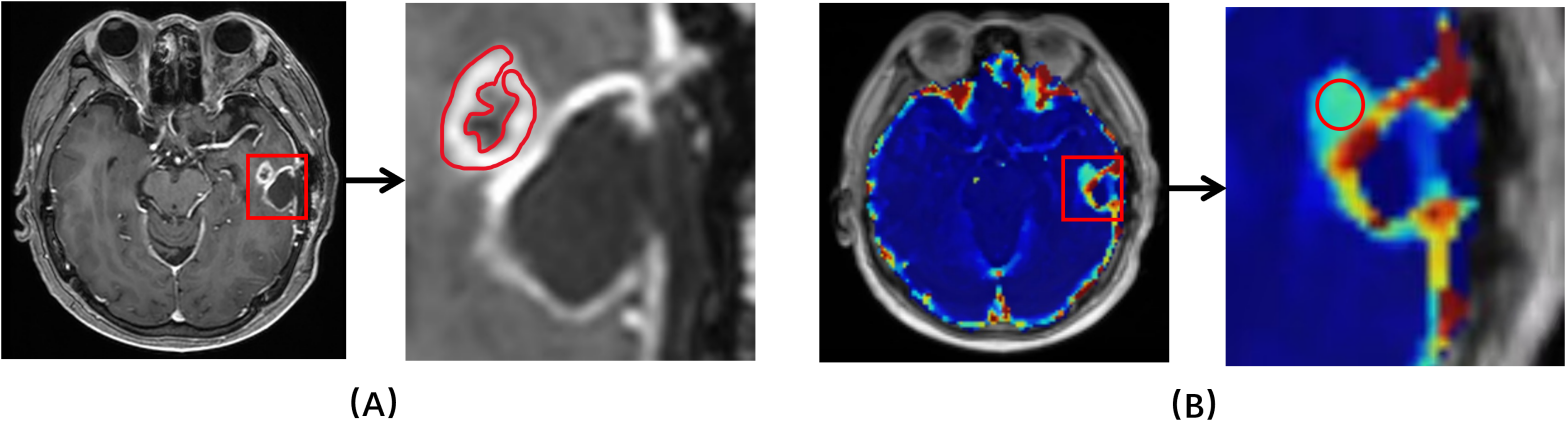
An example of ROIs drawn. (A) Based on structural T1 contrast-enhanced MRI. (B) Based on kinetic parameter maps.
